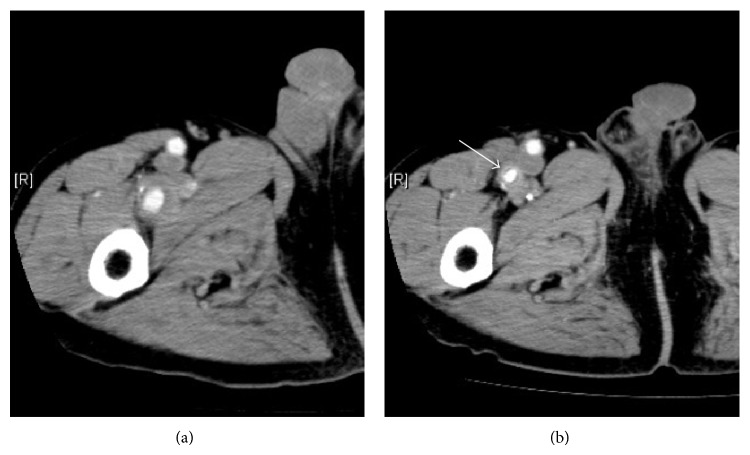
(a) Preoperative computed tomography exhibited a 22 mm right profunda femoris artery aneurysm with an intraluminal thrombus. (b) Postoperative computed tomography revealed a patent replaced prosthetic graft (white arrow).
